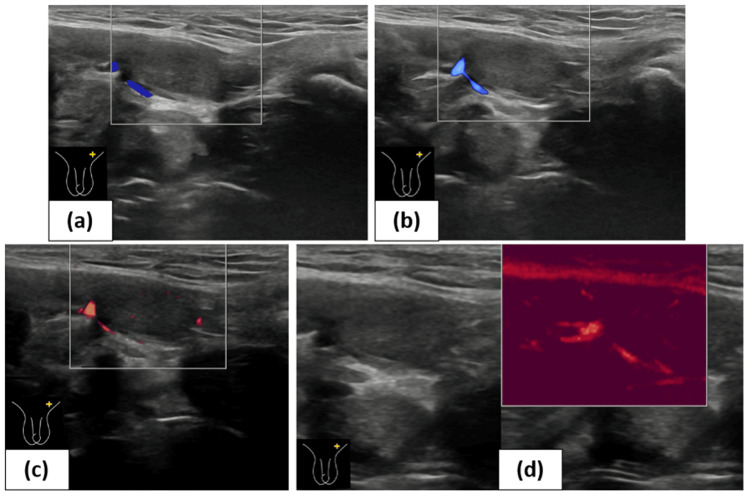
A 18-month-old male patient presented with left inguinal swelling (a) CD shows Grade 0 flow; (b) PD shows Grade 0 parenchymal flow; (c) cMVI shows single spot color encoding signal, i.e., Grade 1 flow; (d) mMVI shows few linear and spot color encoding signals, i.e., Grade 3 flow. CD: Color Doppler; PD: Power Doppler; cMVI: Color Microvascular Imaging; mMVI: Monochrome Microvascular Imaging
